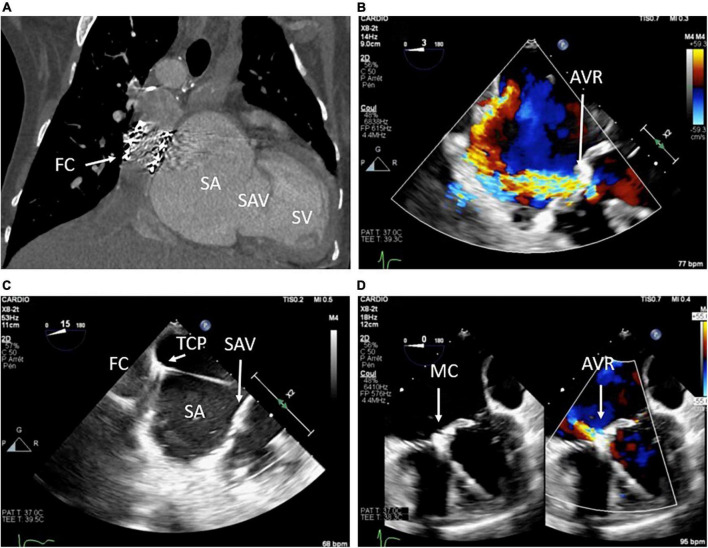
Percutaneous atrioventricular valve edge-to-edge repair procedure in a patient with Fontan Circulation. (A) Computed tomography scanner showing the Fontan conduct (FC) connecting the inferior vena cava to the pulmonary artery, the single atrium (SA), the single atrioventricular valve (SAV), and the single ventricle (SV); (B) transesophageal echography with color Doppler showing the high-grade regurgitation of the atrioventricular valve (AVR); (C) echo guided puncture of the Fontan conduct connecting the inferior vena cava and the left pulmonary artery; (D) result after Mitra-clip (MC) implantation responsible for a dramatic decrease of the AVR.
